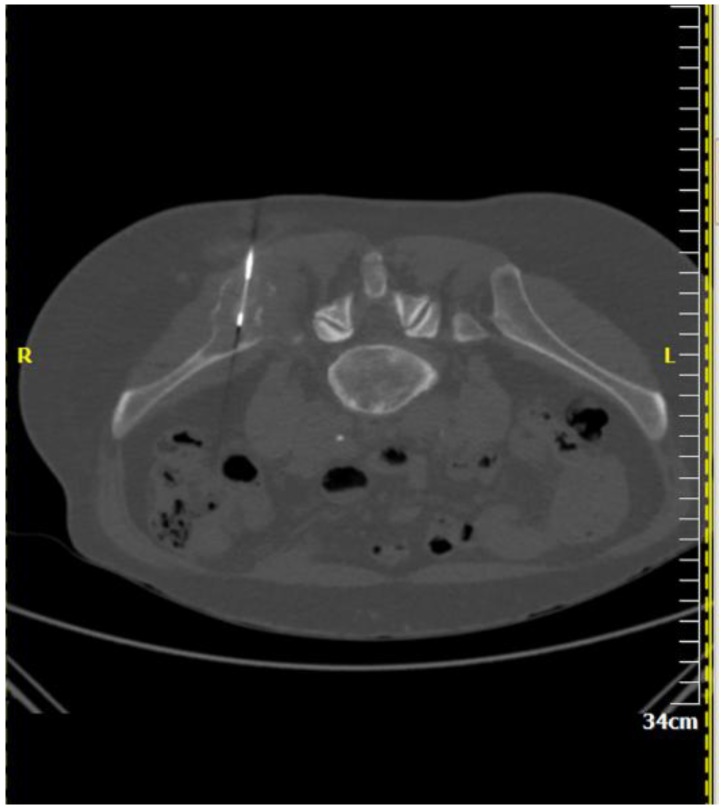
A 64-year-old male patient with multiple osteolytic lesions. Computed tomography axial scan: there is a lytic lesion in the right iliac bone. The result of percutaneous, imaging-guided biopsy was metastasis from small cell bronchogenic carcinoma.
